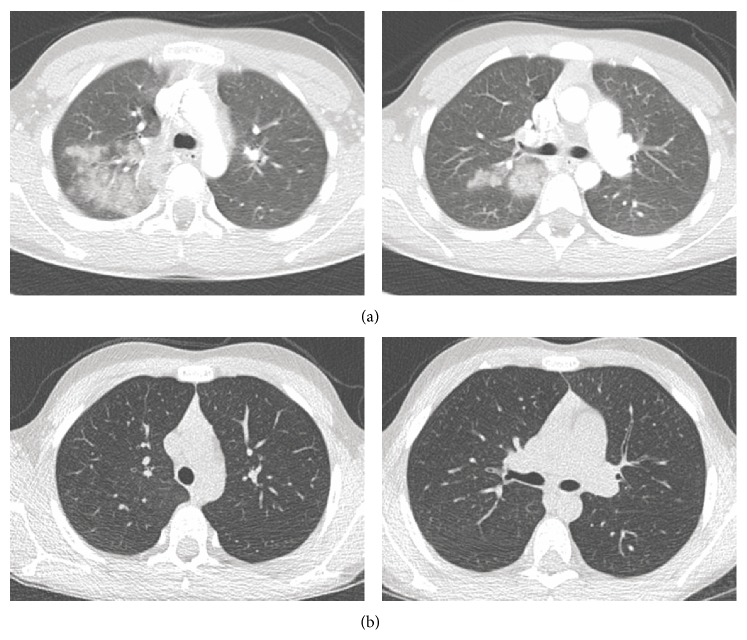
(a) Initial chest CT with upper and lower right lobe consolidations, characterized by low density (−129 HU within the lower lobe consolidation). (b) Chest CT two months later showed complete recovery.
